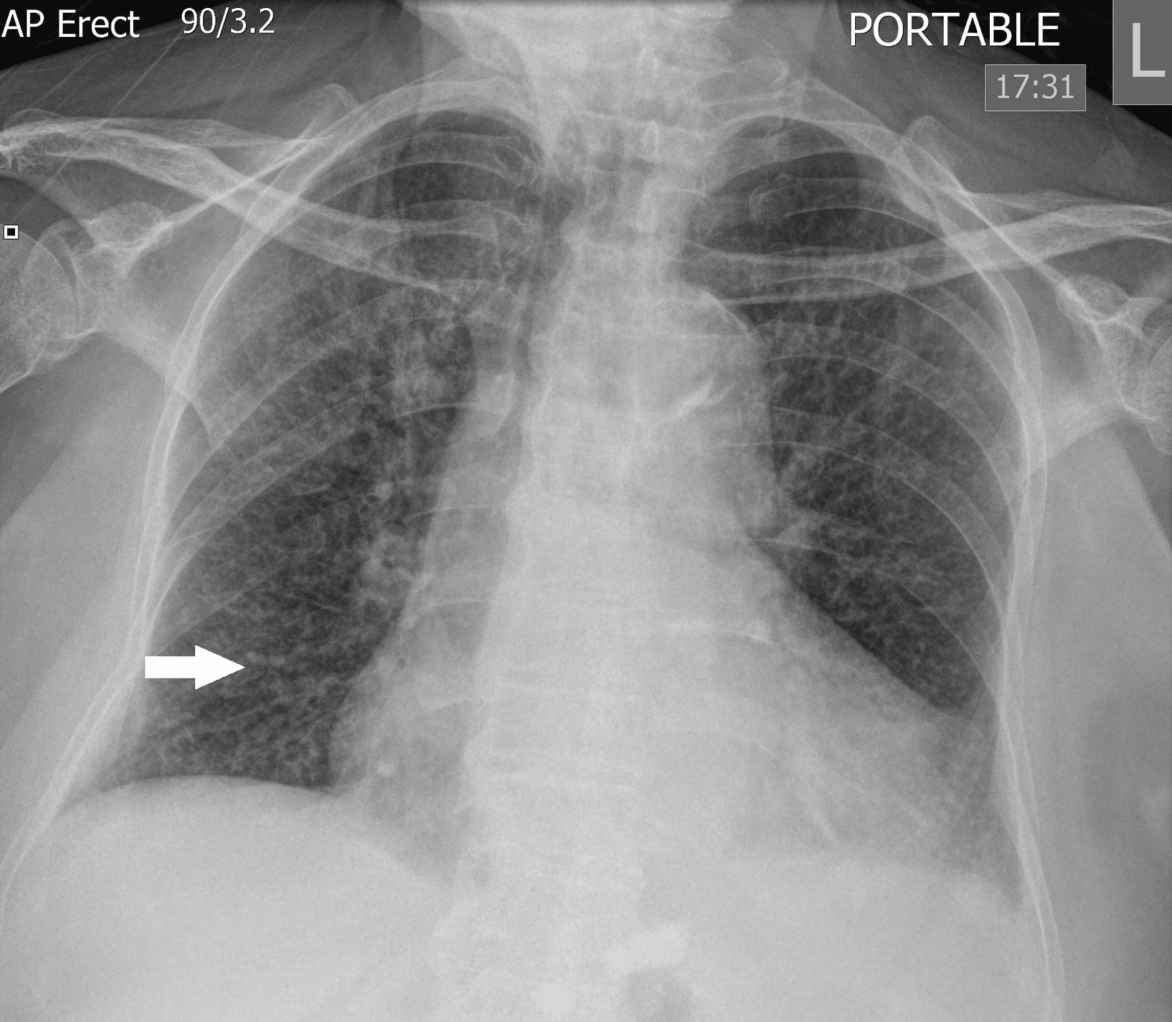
Chest radiograph (AP erect). There are numerous new tiny opacities distributed uniformly in both lungs (white arrow).
